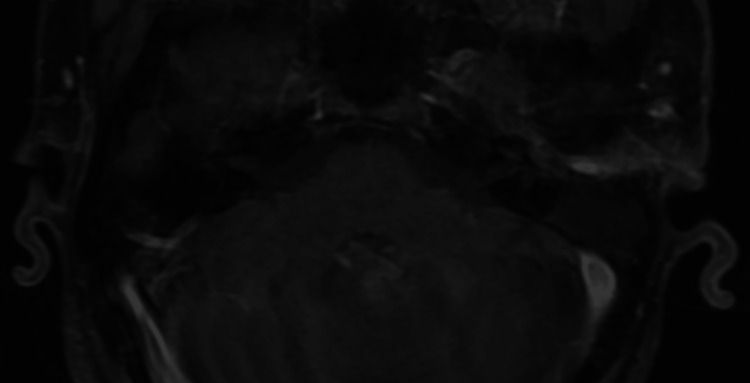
Post-contrast T1-weighted sequence; no enhancement observed in the anterior or posterior portions of the labyrinth
